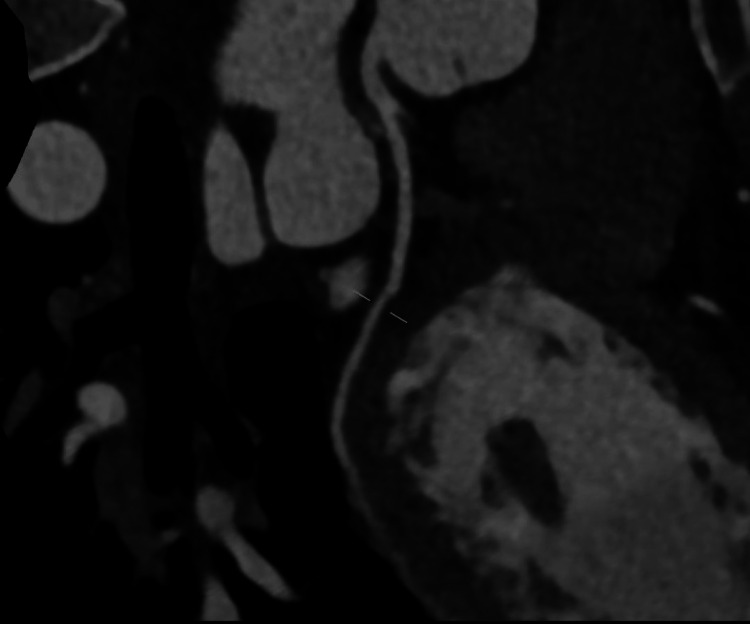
CT coronary angiogram showing patent left circumflex artery
